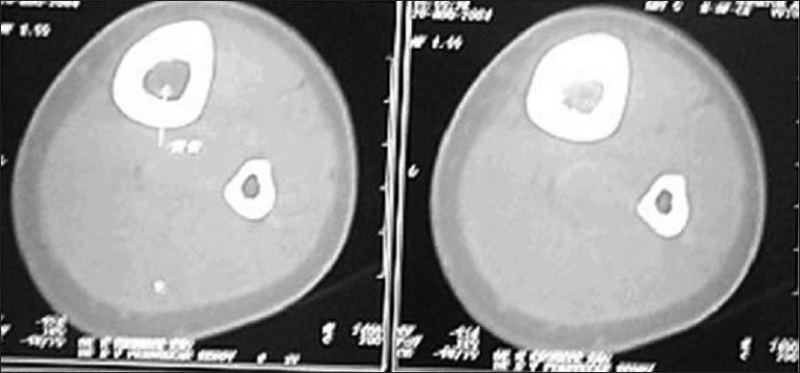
CT of both legs identified sclerosis, but nidus is indefinable
